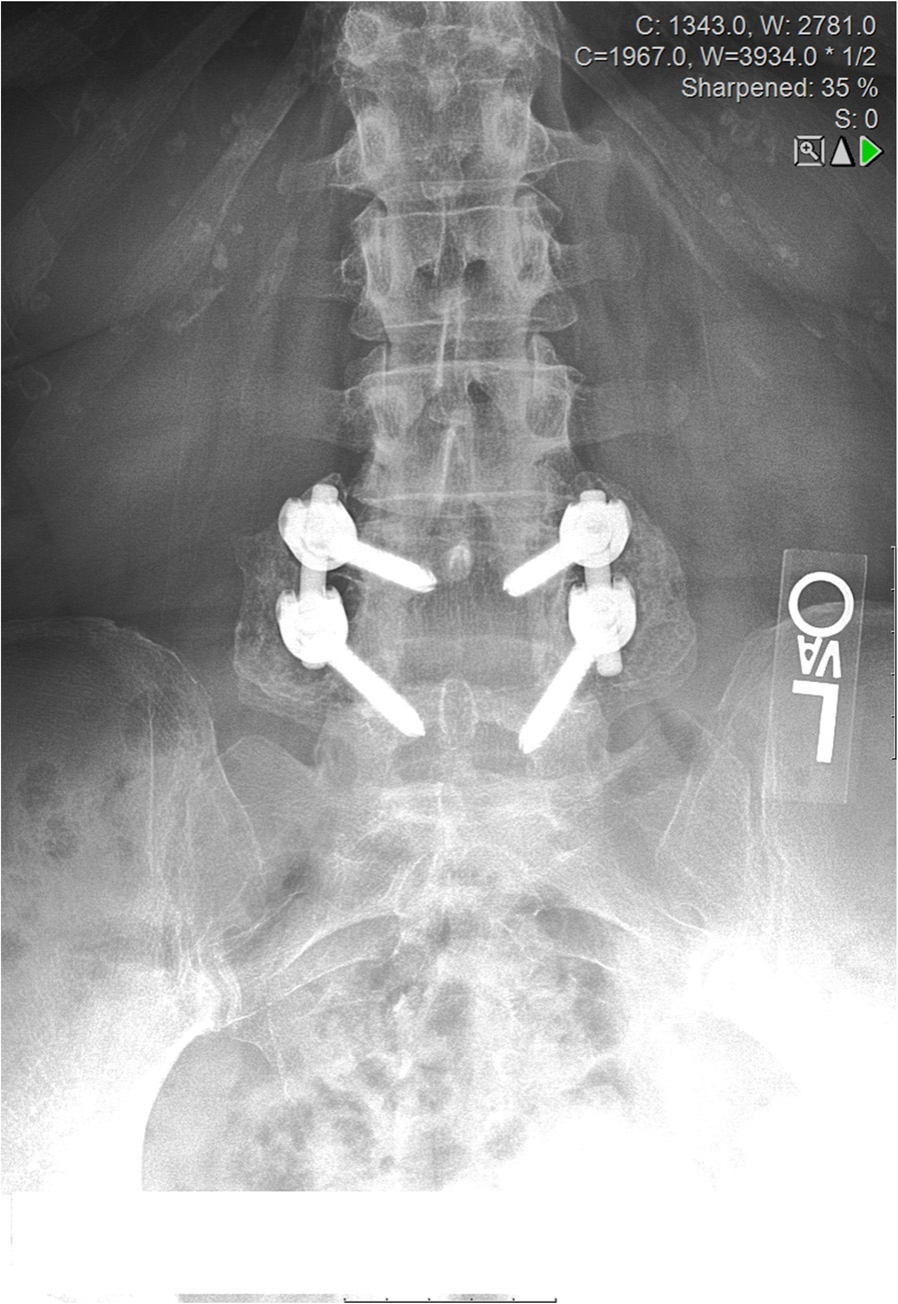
Twelve-month visit AP radiograph. Subject that was diagnosed with grade II degenerative spondylolisthesis and osteoporosis and that was treated with osteoporosis-related medications. Subject received 15 cc TE and 10 cc locally derived autograft. Robust bilateral bone bridging observed at 10.5 months. Patient fell at 6 months postoperatively and obtained a T9 compression fracture, although this apparently had no adverse effect on the fusion mass
